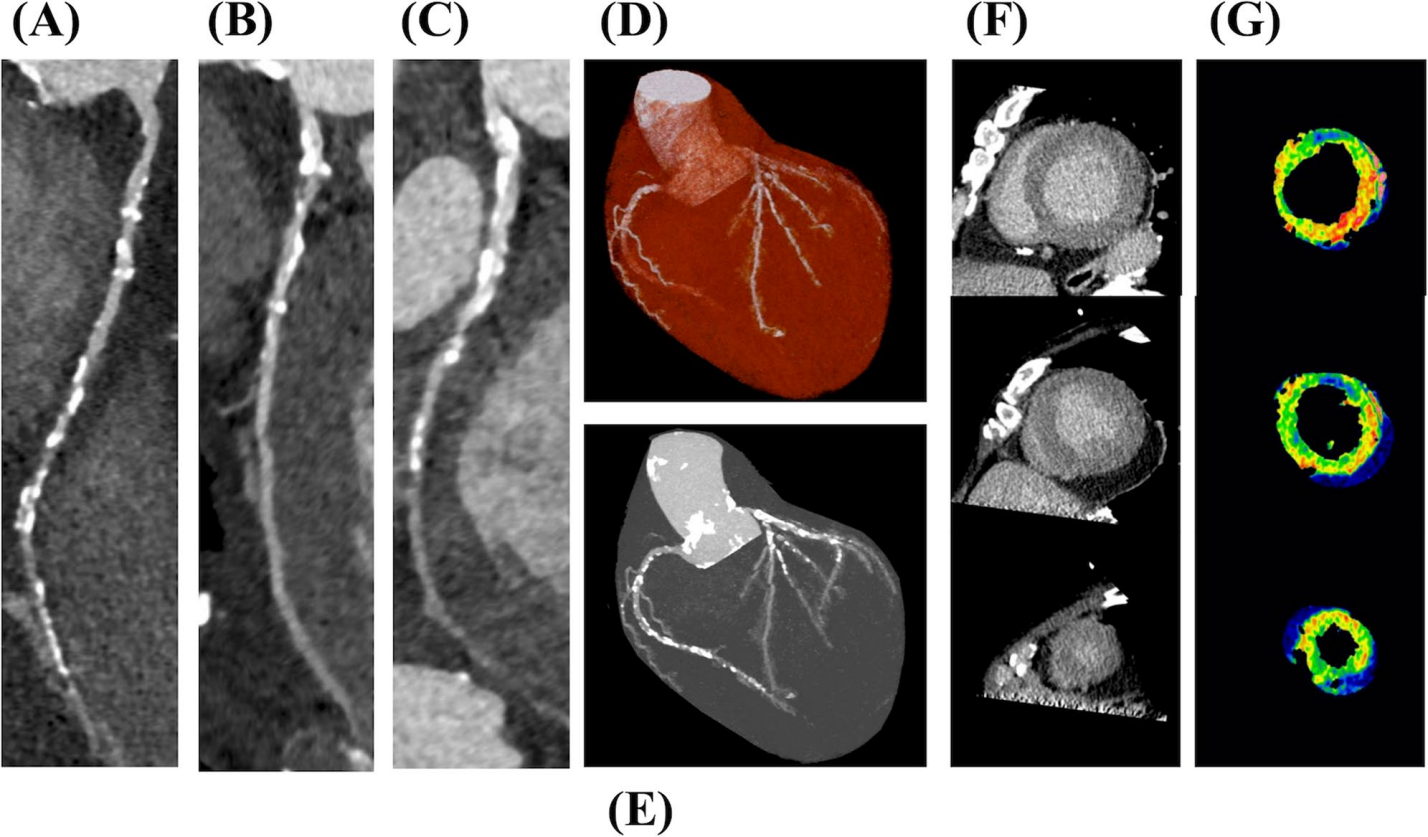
Cardiac CT images of a 70-year-old male who experienced a major adverse cardiac event (MACE). Eleven months after the examination, the patient was hospitalized due to heart failure exacerbation. A Curved multiplanar reconstruction of right coronary artery, B curved multiplanar reconstruction of the left anterior descending coronary artery, C curved multiplanar reconstruction of the left circumflex coronary artery. Multiple moderate to severe stenoses are present in each vessel, with a CAD-RADS score of 4A, indicating obstructive CAD and a calcium score of 3340, D volume rendering image of the heart and coronary arteries, E maximum intensity projection image of the heart and coronary arteries, F delayed enhancement images from the base, mid, and apex, and G MBF maps from base, mid, and apex. The global MBF of the patient was 80.1 ml/g/min; *MACE* major adverse cardiac event, *CAD-RADS* Coronary Artery Disease-Reporting and Data System, *CAD* coronary artery disease, *MBF* myocardial blood flow
